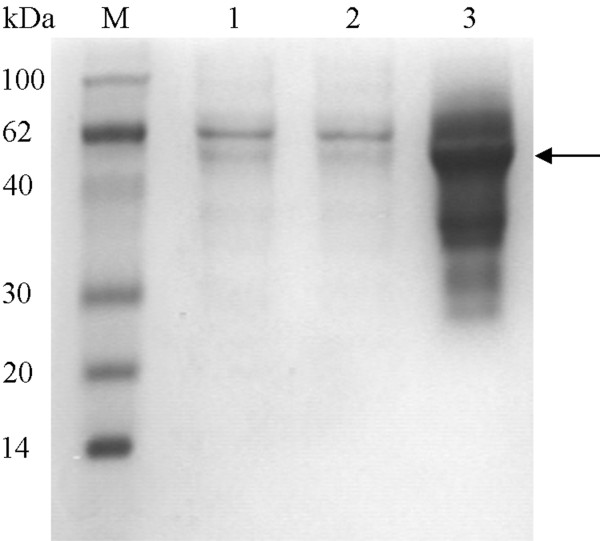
Solubilization of PCPEL2 expressed by pET system. Lane M, molecular mass marker; lanes 1 and 2, the supernatant of fusion protein after ultrasonication at pH 7.0 and 9.0 buffer (10 mM Tris-HCl, 150 mM NaCl); lane 3, the supernatant of fusion protein after ultrasonication with suspending buffer (10 mM Tris-HCl pH 10.3 containing 150 mM NaCl, 2 M urea, 10 mM imidazole and 5 mM β-mercaptoethanol). The over-expressed protein is indicated by the arrow.
